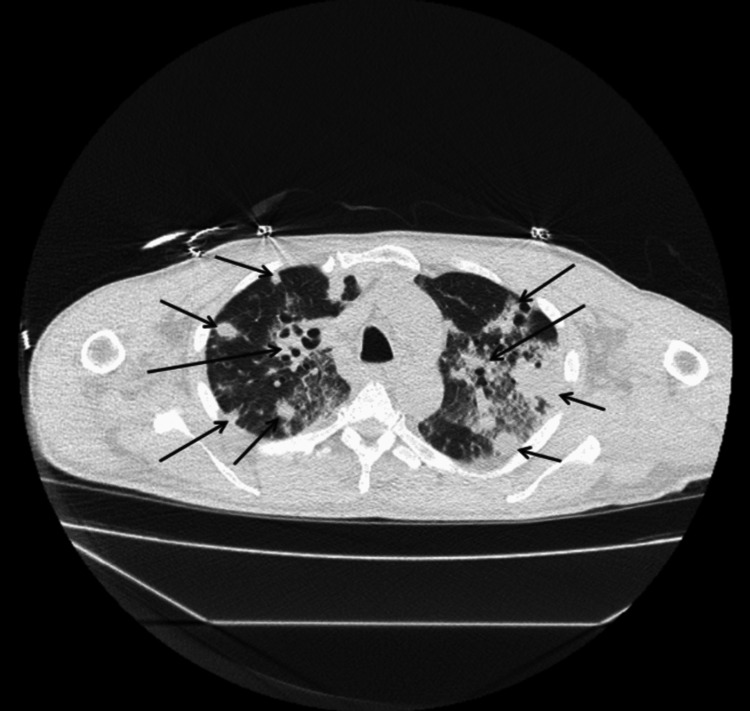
CT chest axial view without contrast showing upper parts of both lungs. The arrows show multiple, bilateral diffuse airspace cavitations with air bronchograms in some cavities, consistent with septic pulmonary emboli.
